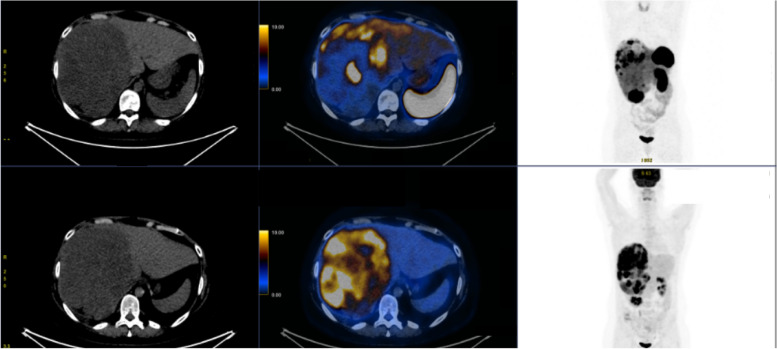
^68^Ga-DOTATOC PET/CT scan showing a bulky adrenal mass and liver metastasis
